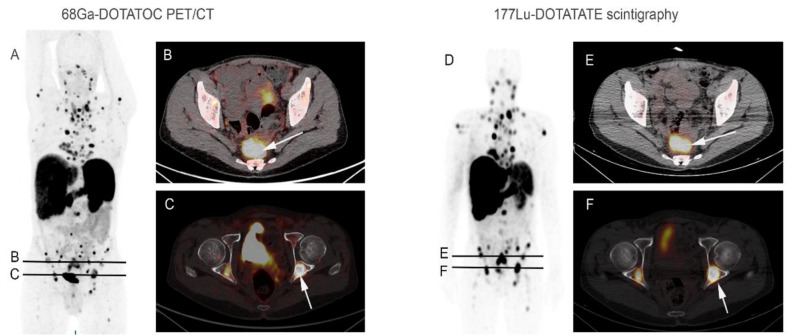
A 65-year-old man with metastatic well-differentiated grade 2 rectal neuroendocrine tumor, progressive after chemotherapy, addressed for PRRT. ^68^Ga-DOTATOC PET/CT maximum intensity projection image (A) and axial fused PET/CT images (B,C) showed high multiple focal uptakes, corresponding to mediastinal, abdominal lymph nodes, liver and bone lesions ((C) arrow: example of bilateral cotyle posterior wall lesions) associated with a large rectal lesion ((B) arrow). Post ^177^Lu-DOTATATE therapy whole-body image (D) after the first administration showed focal tracer uptake in all lesions correlating with ^68^Ga-DOTATOC PET/CT images, as observed in fused SPECT/CT images (E,F).
